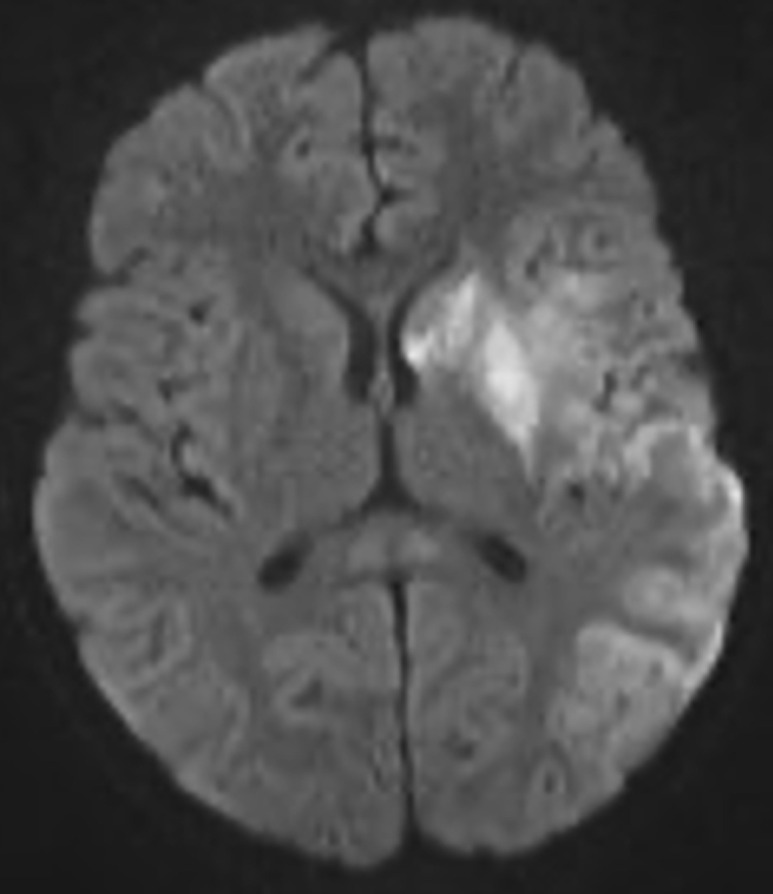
diffusion axial slice of MRI brain showing areas of restriction on which are involving caudate nucleus, putamen, insular cortex and parietal lobe
